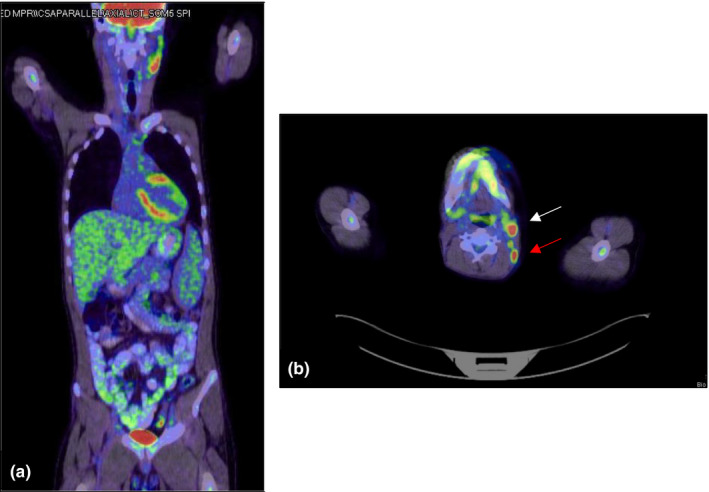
A, Hypermetabolic left cervical lymph node, 1.3 cm in diameter, SUV of 12.2. (White arrow). B, Left posterior neck is also showing few nodes around 7‐9 mm with SUV extending up to 7.7. (Red Arrow)
